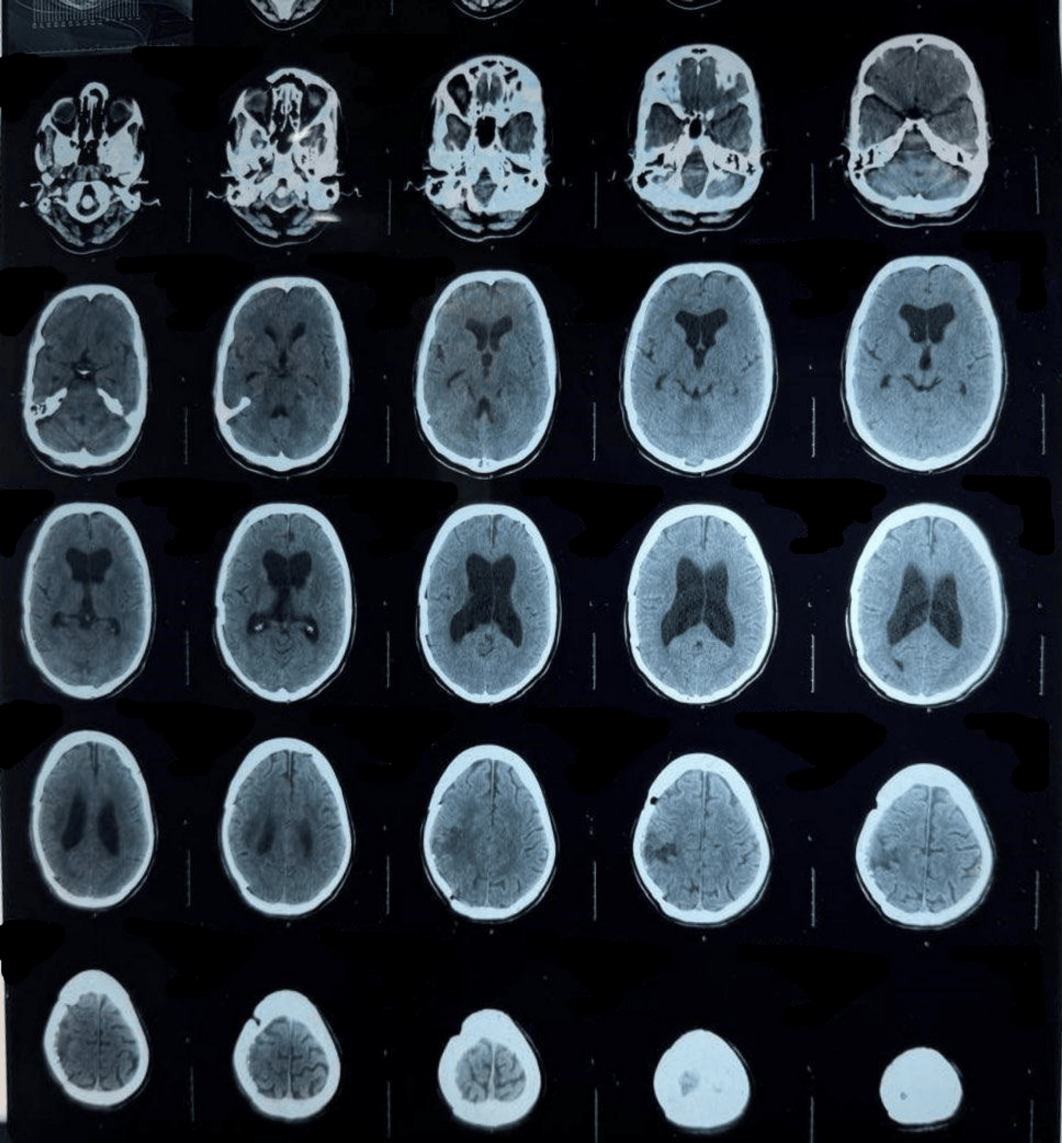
Postoperative CT scan confirming tumor resection and assessing post-surgical changes
